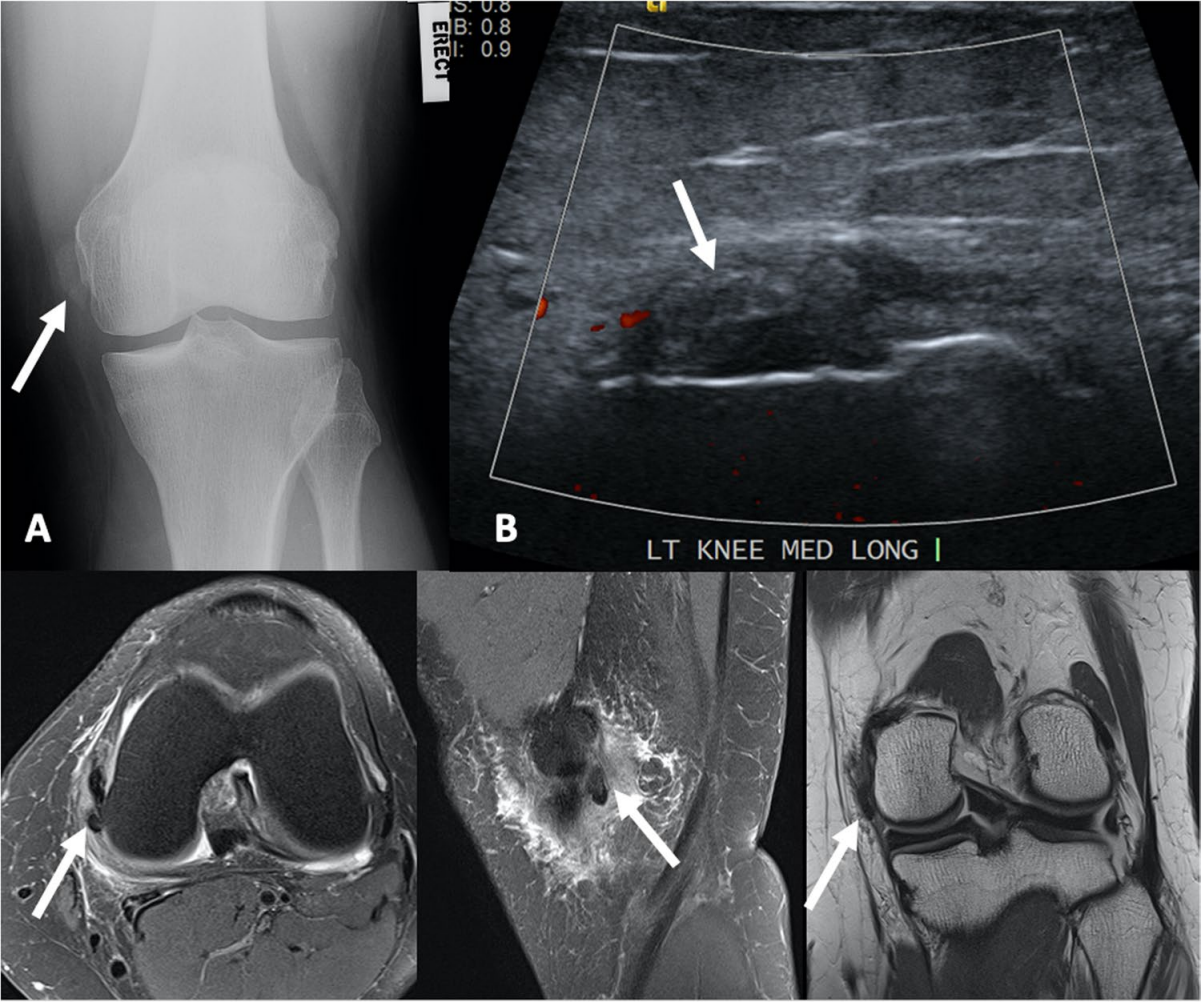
A 51-year-old female presented after a sudden onset of medial knee pain, 1 day after weight training. A AP weight-bearing radiograph of the left knee demonstrates an ovoid focus of calcification (arrow) adjacent to the medial condyle of the femur. B Longitudinally orientated ultrasound image of the medial aspect of the knee with color Doppler also shows the calcification in continuity with the posteromedial tibiofemoral joint capsule (arrow) and mild adjacent hyperemia. An MRI was performed 3 days after the plain film and ultrasound studies. C Axial proton density with fat saturation, D sagittal proton density with fat saturation and E coronal proton density show the capsular calcification (arrow) posterior to the medial collateral ligament, with moderate adjacent soft tissue edema
